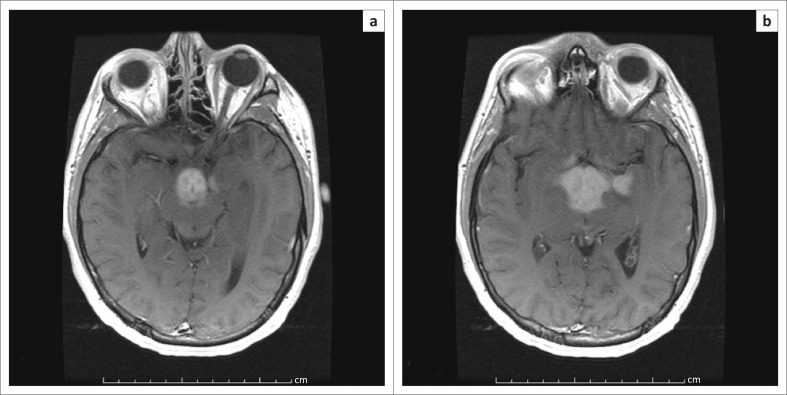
Axial T1-weighted post-contrast imaging (a and b) demonstrating avid enhancement corresponding to the regions of T2/FLAIR signal abnormality.
